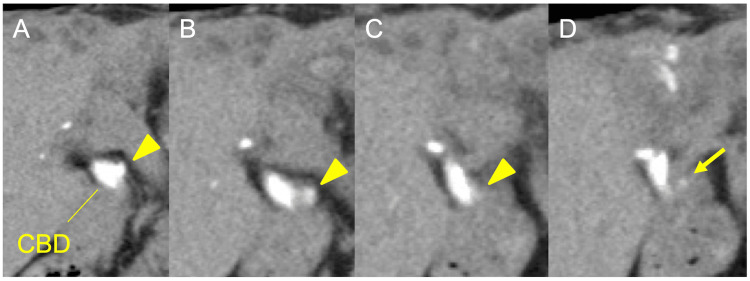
Surgical clip at the cystic duct stump. A-D. Based on the previous drip infusion cholangiography–computed tomography, the remnant cystic duct (arrowhead) is seen flowing into the common bile duct. A surgical clip (Hem-o-Lok, size M) marks the cystic duct stump (arrow). These coronal images are presented in consecutive slices. CBD, common bile duct
